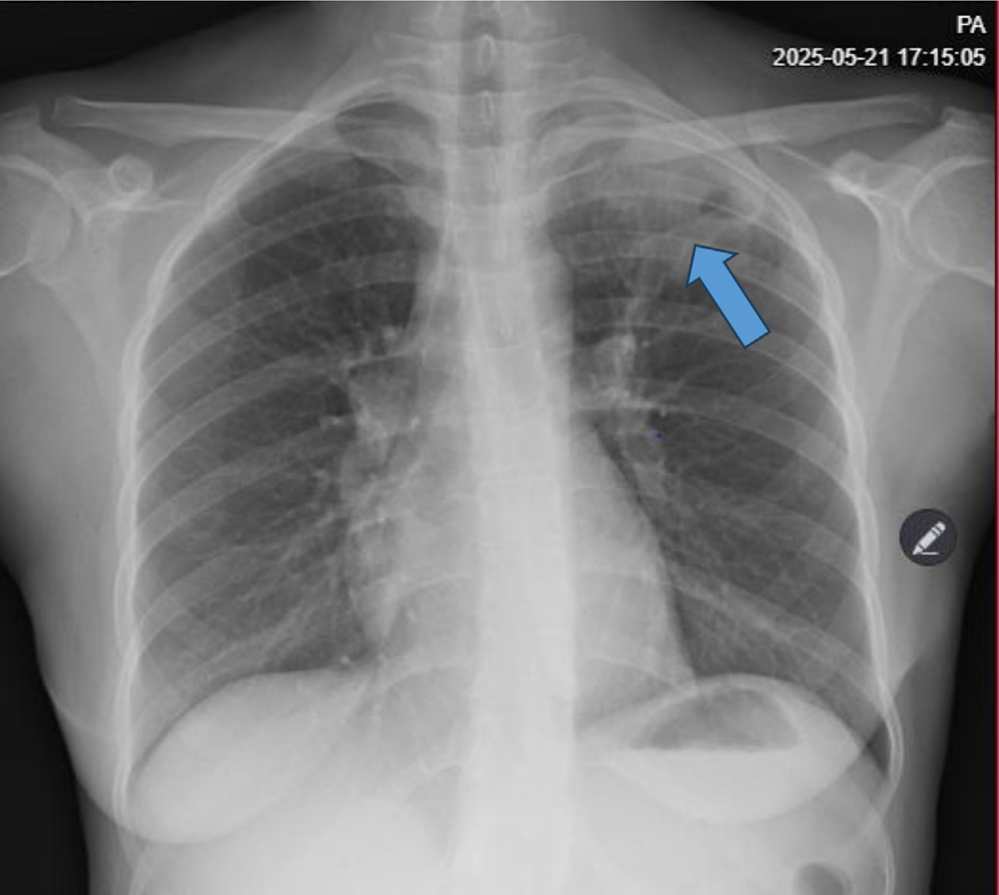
PA chest X-ray showing a homogeneous opacity in the left upper lung zone (blue arrow) with a suspicious cavitary component suggestive of pulmonary tuberculosis.
